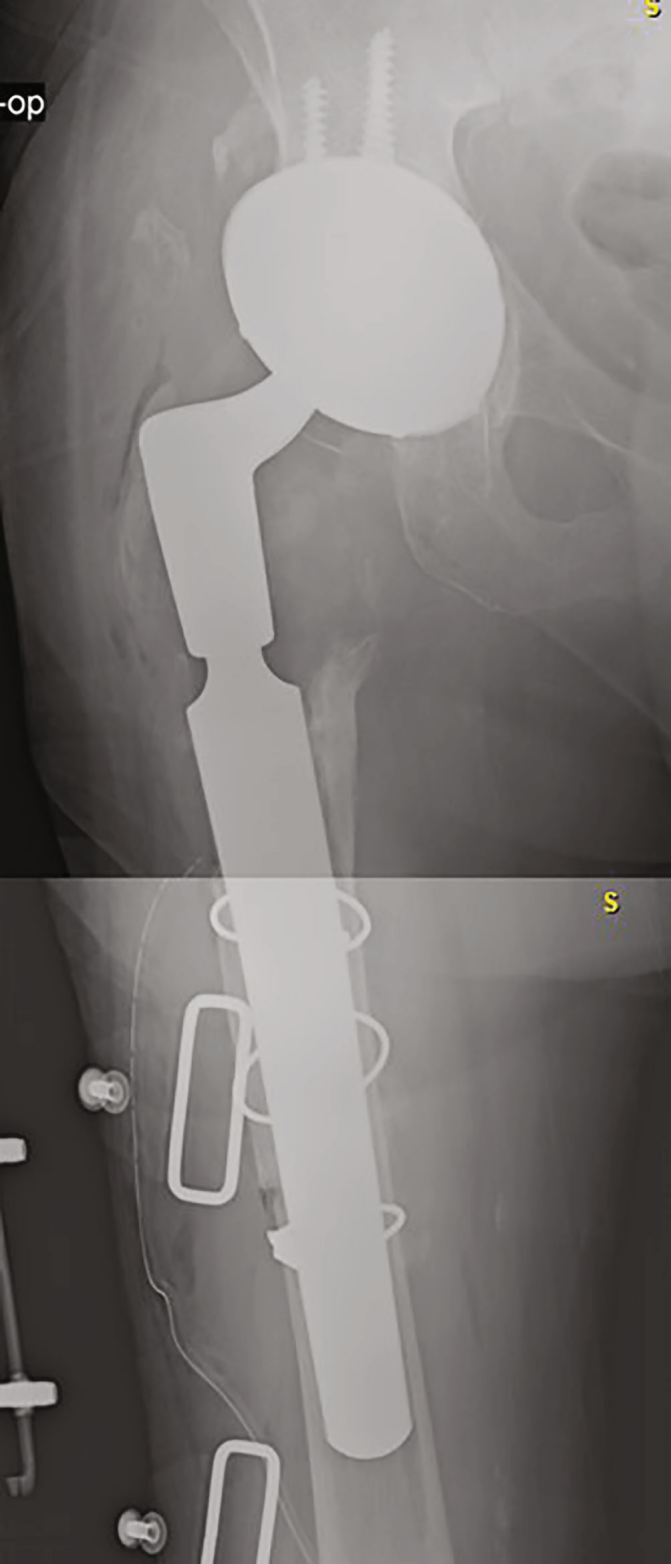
Anteroposterior X-ray of the right hip after reimplantation before fixation.
